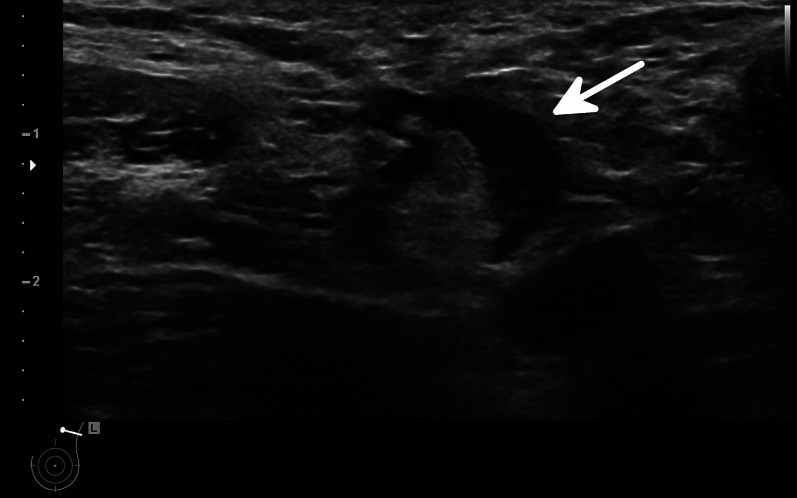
Ultrasonography of the left axilla showing an enlarged 17 mm reactive lymph node in a 45-year-old woman about a week after receiving the first dose of the Vaxzevria COVID-19 vaccine. Note the asymmetrical cortical thickening (white arrow) associated with a well-represented central fatty hilum
